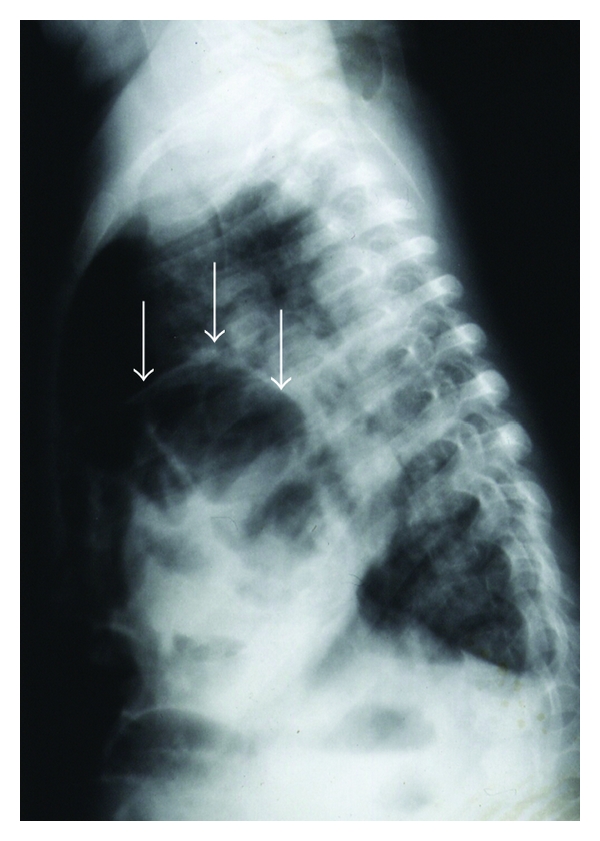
Lateral chest X-ray showing bowel herniation into the chest.
